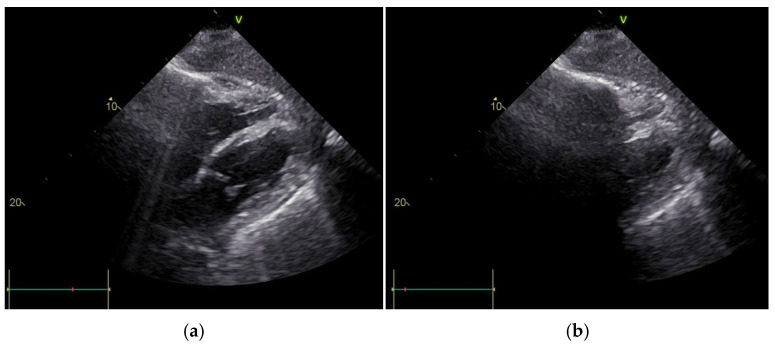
Transthoracic echocardiography subxiphoid view showing a cyclic loss of echogenicity with cardiac structures visible in diastole (a) and disappearing during systole (b).
